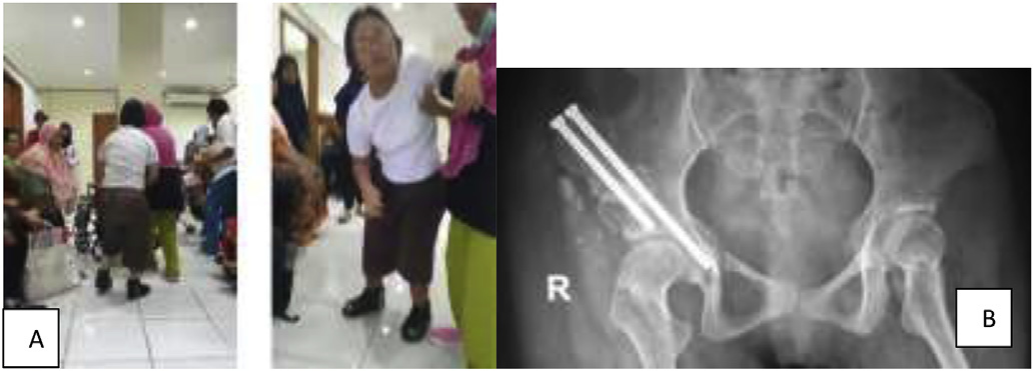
Post-operative clinical examination and radiograph after 5 years follow up. A, clinical ability to stand full weight bear with aid; B, 5 years post-op radiograph showing union at osteotomy sites, and concentric hip.
